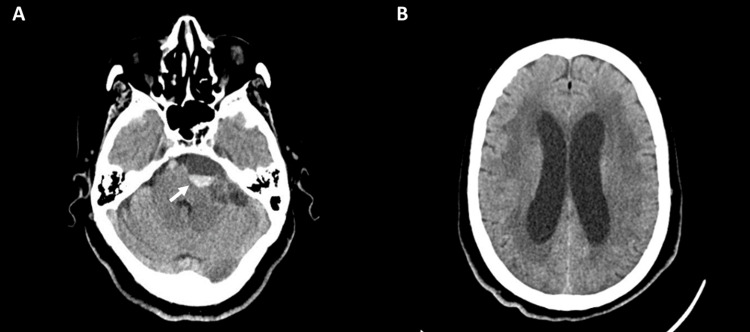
(A,B) Axial cranial CT revealing extra-axial space-occupying lesion (arrow) in the left angle-cerebellar cistern without other lesions.
